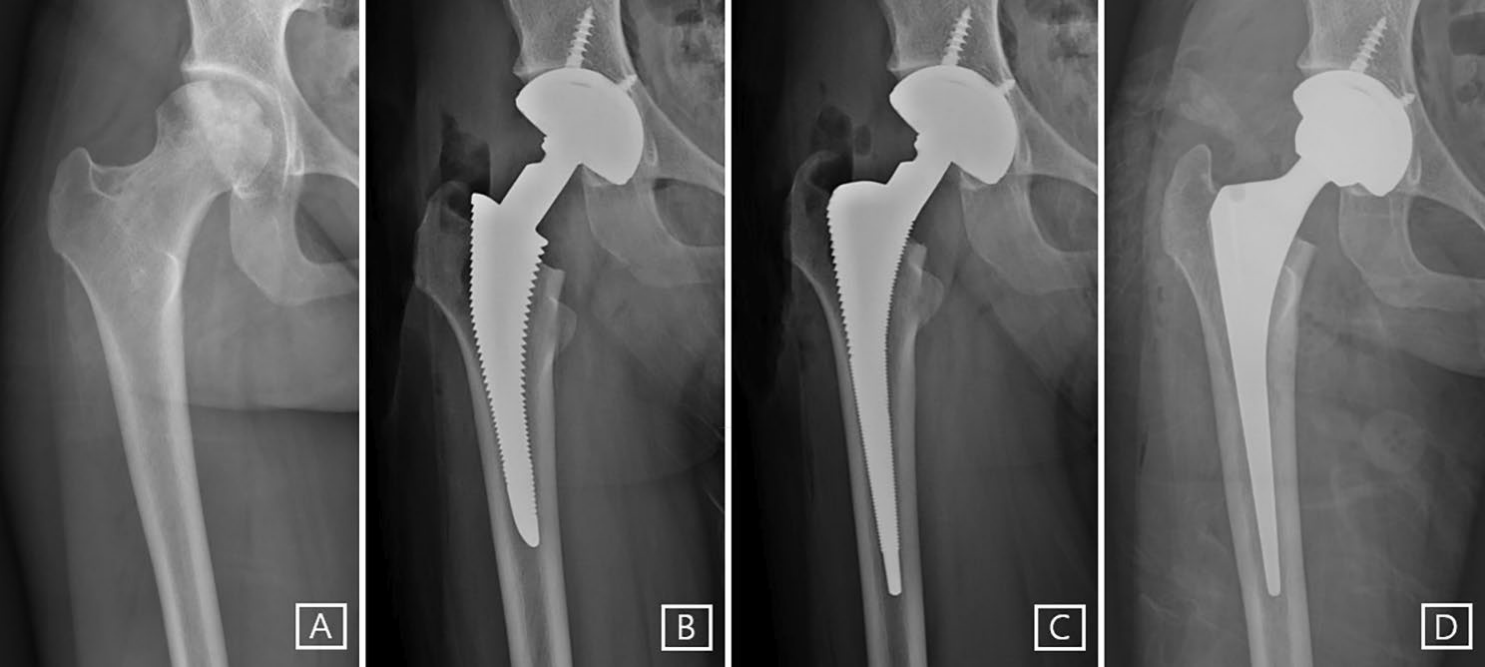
(A) A preoperative anteroposterior radiograph of 24-year-old woman with an osteonecrosis of the femoral head. She had a Dorr A femur, and we initially decided to use the smallest type 1 stem through preoperative templating. (B) In the intraoperative radiograph, the trial of the smallest type 1 stem could not be fully inserted and distal part of the stem was captured by narrow femoral canal due to severe metaphyseo-diaphyseal (M-D) mismatch. (C) In the consequent intraoperative radiograph, the trial of type 3 C stem was fully inserted and properly located. (D) The postoperative radiograph showed properly located and stably fixed implants
